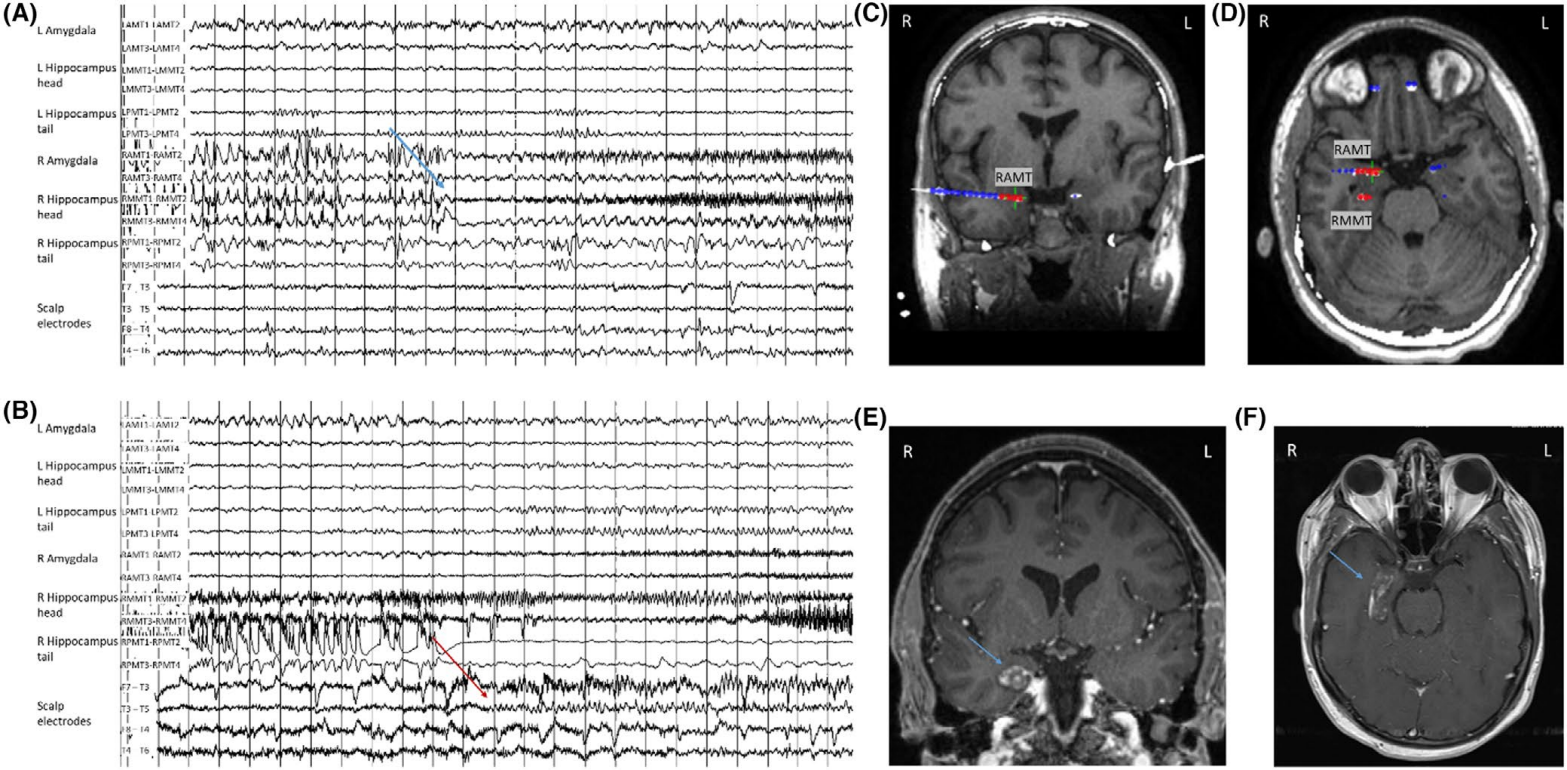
Ictal SEEG (A, B), reconstructed electrodes (C, D), and surgical outcome (E, F) of the patient discussed in Figures 9 and 13. The SEEG page shown in A (25 s, LFF 1 Hz, HFF off, modified bipolar montage with selected channels for clarity) demonstrates pre‐ictal repetitive spiking followed by ictal onset characterized by low voltage fast activity (arrow) over the hippocampal head (RMMT 1–2) closely followed by right amygdala (RAMT 1–2). The page shown in B demonstrates scalp EEG onset characterized by rhythmic 6 Hz theta activity over the left temporal region, which is noted 70 s after SEEG ictal onset, and correlates with ictal propagation to the left hippocampus on SEEG (LPMT) characterized by rhythmic 6‐7 Hz theta activity. Electrodes were visualized by image co‐registration between a pre‐implantation T1‐weighted volumetric MRI and a post‐implantation volumetric CT using the Brainlab software and Curry 8 software, with most active contacts at seizure onset highlighted in red (C, D). These findings confirmed a right mesial temporal lobe epilepsy and suggested that the seizures seen on scalp EEG were falsely lateralizing. The patient opted for right mesial temporal laser interstitial thermal therapy (LiTT) and the ablation zone is shown in E and F. He remains seizure‐free with an Engel IA outcome at 5 years. The first letter specifies laterality: R (right) or L (left); the second letter specifies the anterior–posterior position: A (anterior), M (middle), or P (posterior); the third letter specifies the superior–inferior position: S (superior), M (middle), or I (inferior); the fourth letter specifies the lobe: F (frontal), T (temporal), P (parietal), or O (occipital). OF indicates the orbitofrontal region. Example, “RAMT” refers to the right anterior middle temporal region.
